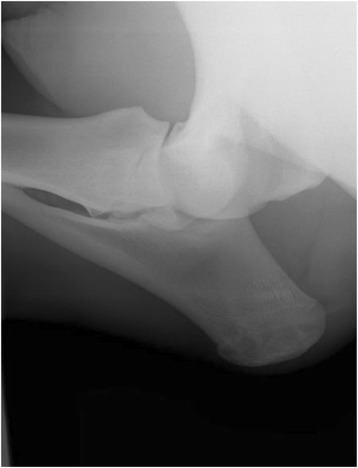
Mediolateral radiographic views of the elbow of a 3 year old thoroughbred gelding with lateral partial avulsion of the ulnaris lateralis and enthesiopathy of the lateral epicondyle of the humerus. No radiographic abnormalities were identified
